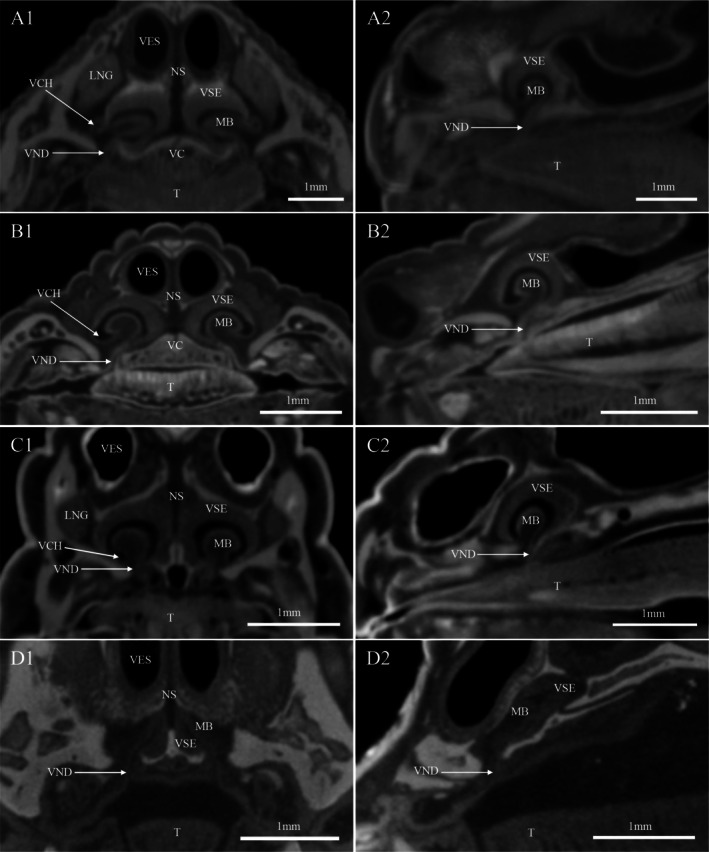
Transverse and sagittal CT scans of the vomeronasal organ of four species of lizards. (A1, A2) *T. roborowskii* (active foraging); (B1, B2) *T. dadunensis* (sit‐and‐wait foraging); (C1, C2) *E. roborowskii* (active foraging); (D1, D2) *P. axillaris* (sit‐and‐wait foraging). LNG, lateral nasal gland; MB, mushroom body; NS, nasal septum; T, tongue; VC, vomerine cushion; VCH, ventral channel; VES, vestibulum; VSE, vomeronasal sensory epithelium; VND, VNO duct.
